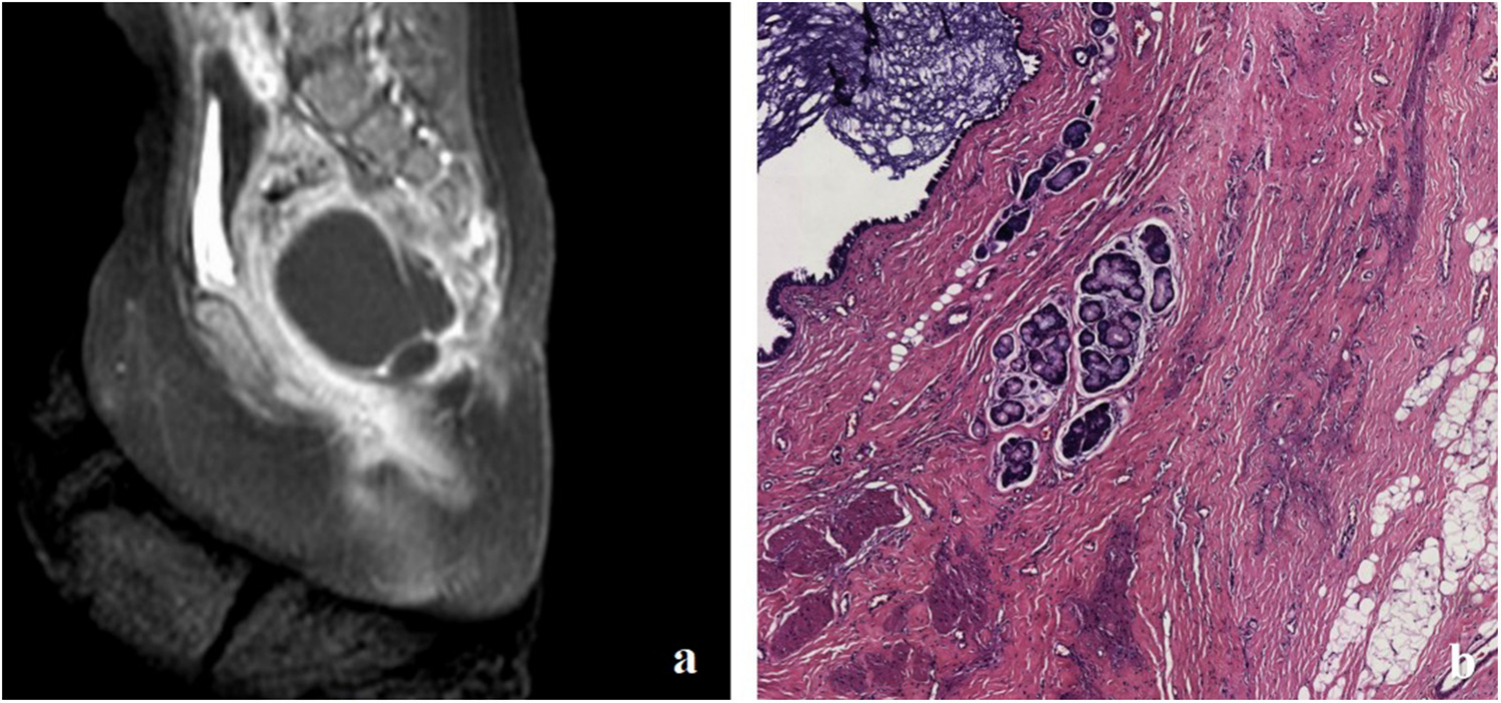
(a) MRI showing a cystic lesion located anterolaterally to the sacrococcygeal area in case 1. (b) Postoperative pathology confirmed the diagnosis of a mature cystic teratoma.
